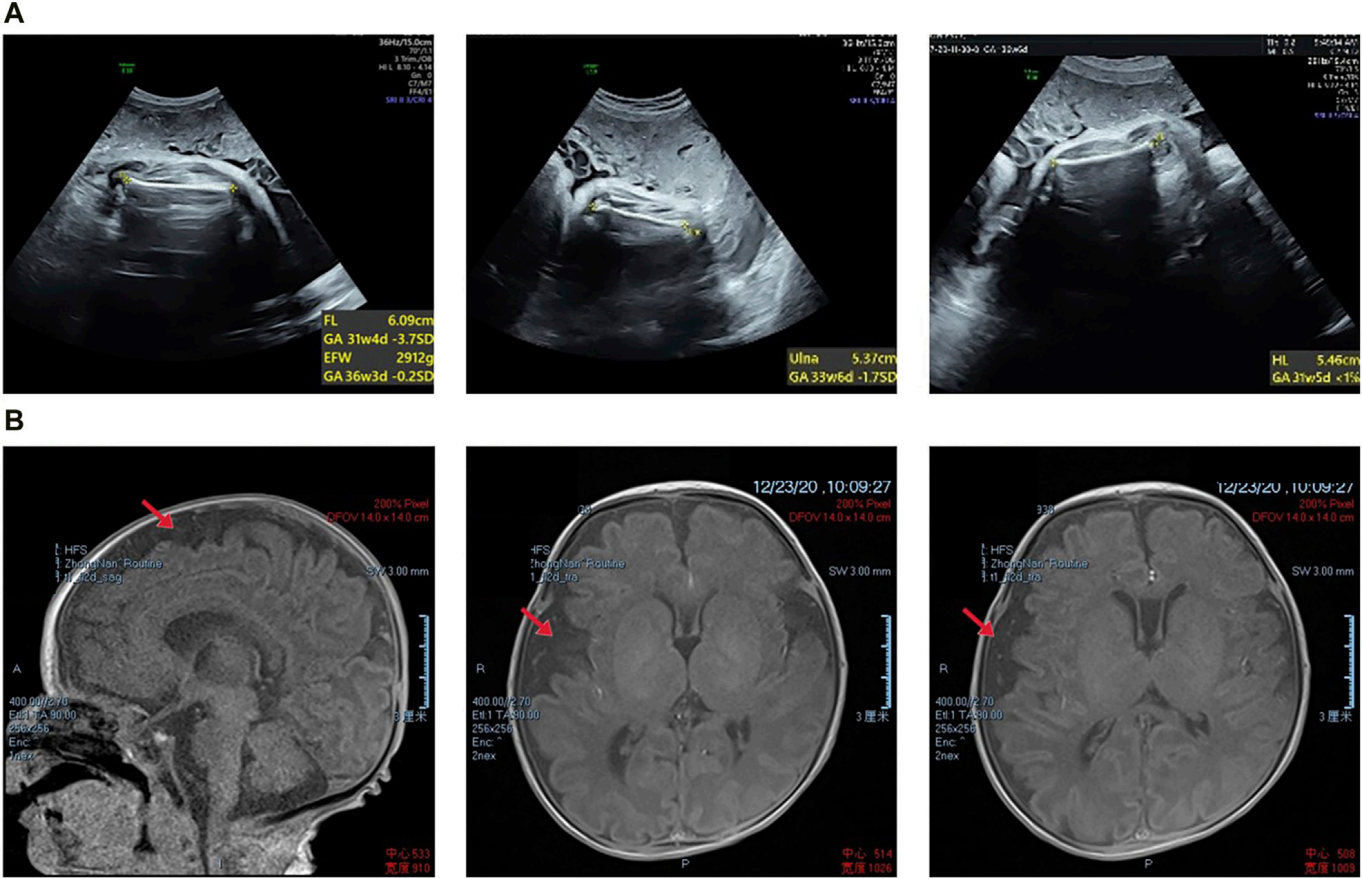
(A) Prenatal transabdominal ultrasound images were taken of the fetus at 31^+4^ weeks’ gestation, which showed short limbs. (B) The proband’s magnetic resonance imaging results revealed slight asymmetry in the bilateral brain parenchyma, wider gyri, and a slight widening of the subarachnoid space in the right frontal, parietal, and temporal lobes. Additionally, there was a slight leftward tilting of the midline structure.
